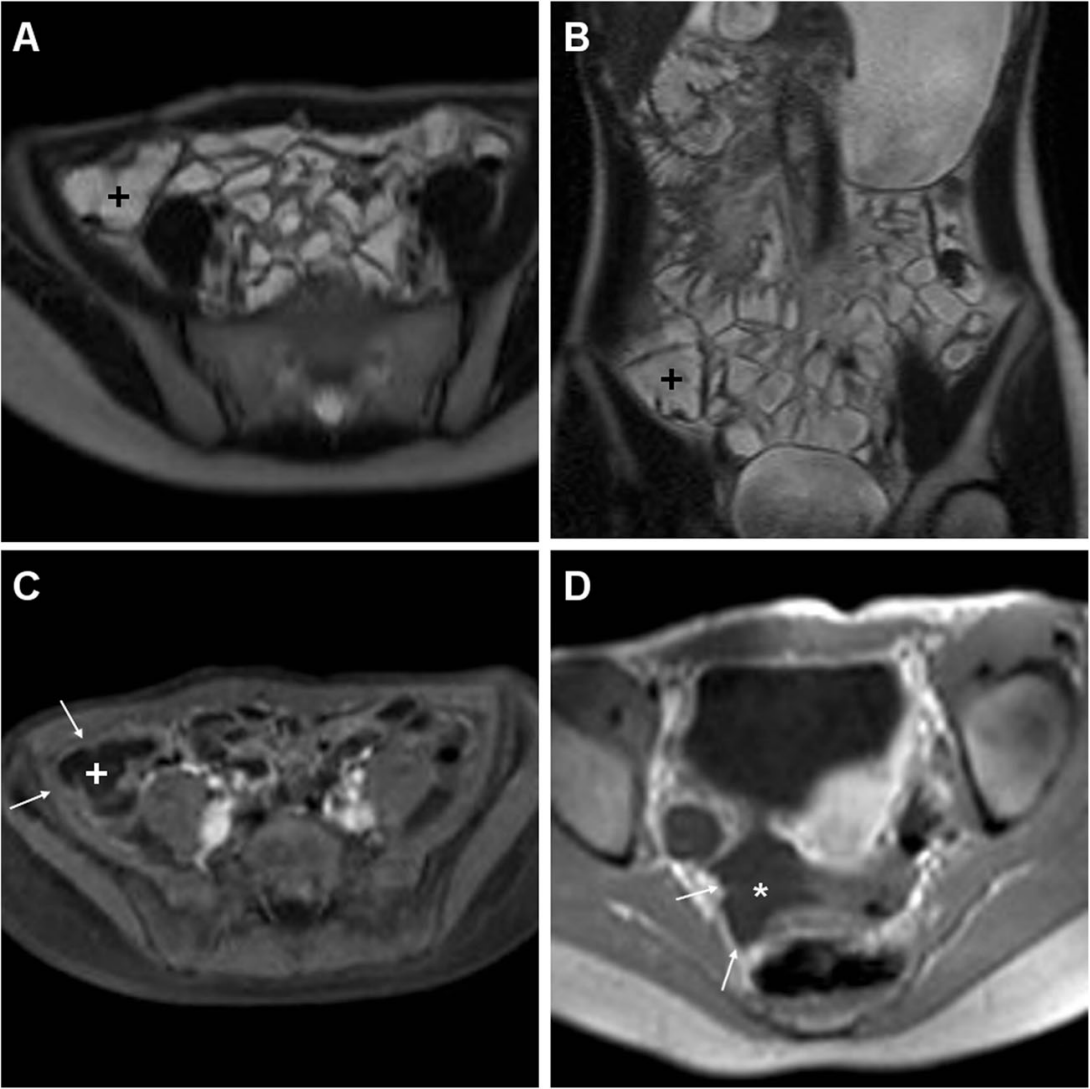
A 16-year-old female with intermittent low-grade fever, failure to thrive and weight loss 15 weeks after LA for uncomplicated AA. Considering the patient’s young age, to avoid use of ionising radiation investigation was carried out by means of MR enterography including peroral bowel distension with diluted polyethylenglycol solution. Axial (a) and coronal (b) T2-weighted images show optimal ileal and caecal (+) distension by intraluminal fluid, without residual inflammatory fat stranding and appreciable abscess collection. After intravenous gadolinium-based contrast, T1-weighted images with (c) and without (d) fat suppression exclude abnormal enhancement of the peritoneal serosa (*thin arrows*), with minimal fluid (* in d) in the peritoneal cul-de-sac. The patient did not require further treatment
